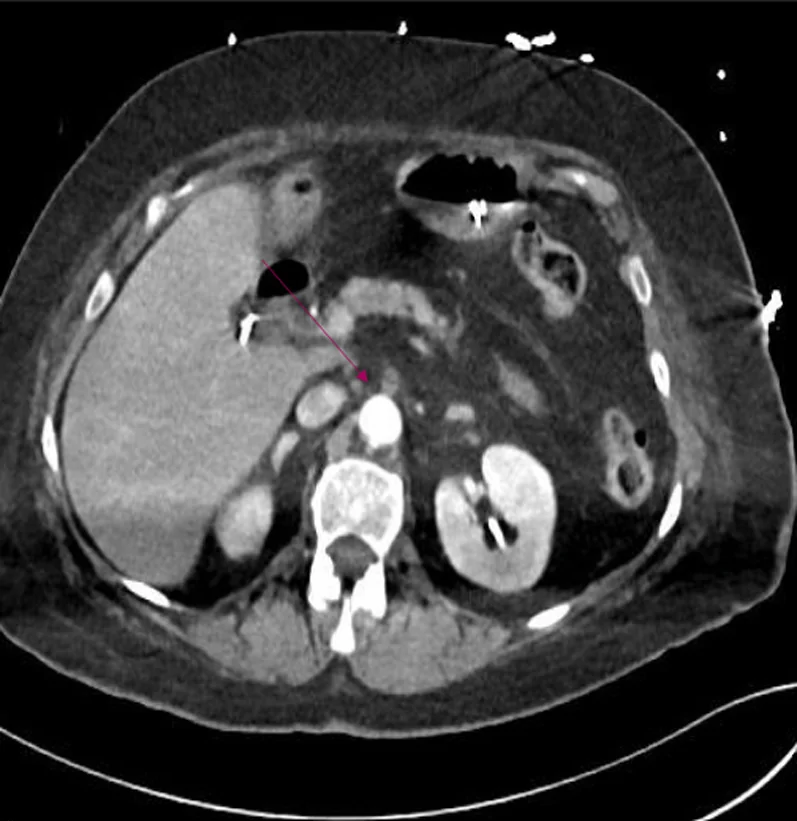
Terminal Aorta Bifurcation and Lower Extremity Runoff Axial computed tomography angiography slice obtained at the level of the lower lumbar spine showing the terminal abdominal aorta bifurcation. A distinct, noncalcified, low-attenuation filling defect is visible within the lumen of the right common iliac artery (arrow), representing acute embolic entrapment.
